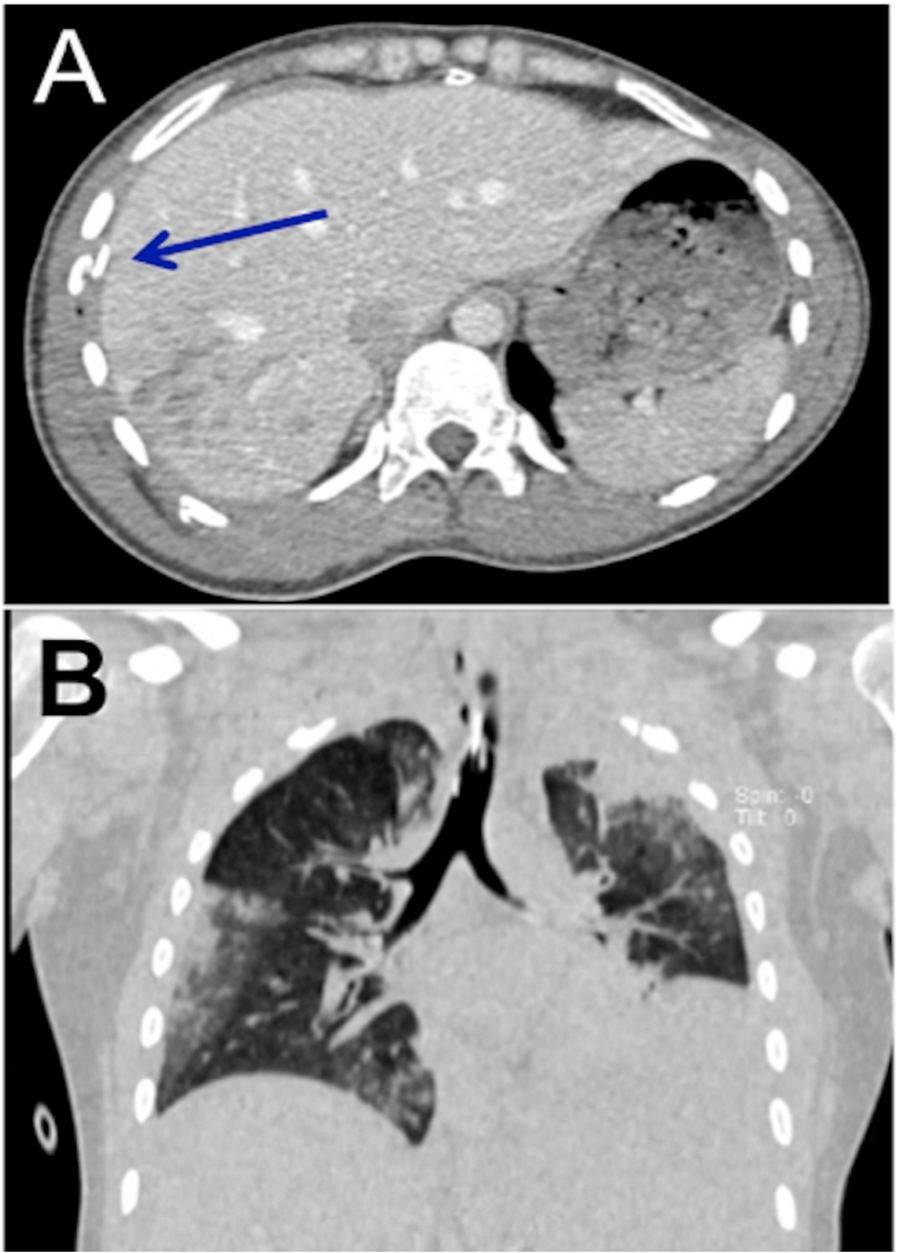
Chest CT in axial cross-section showing right-sided rib fractures (A, arrowed) and coronal section showing lung contusions (B); injuries frequently associated with liver trauma.
